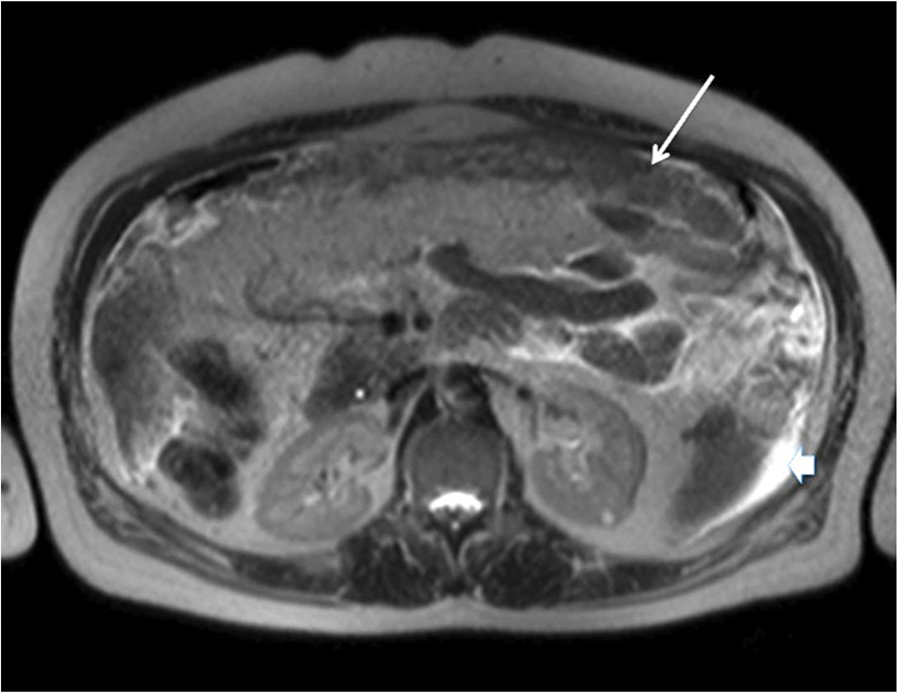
Abdominopelvic magnetic resonance imaging. Axial T2-weighted image. Heterogeneous soft-tissue thickening of the greater omentum (“omental cake”) (arrow). Moderate ascites (arrowhead).
